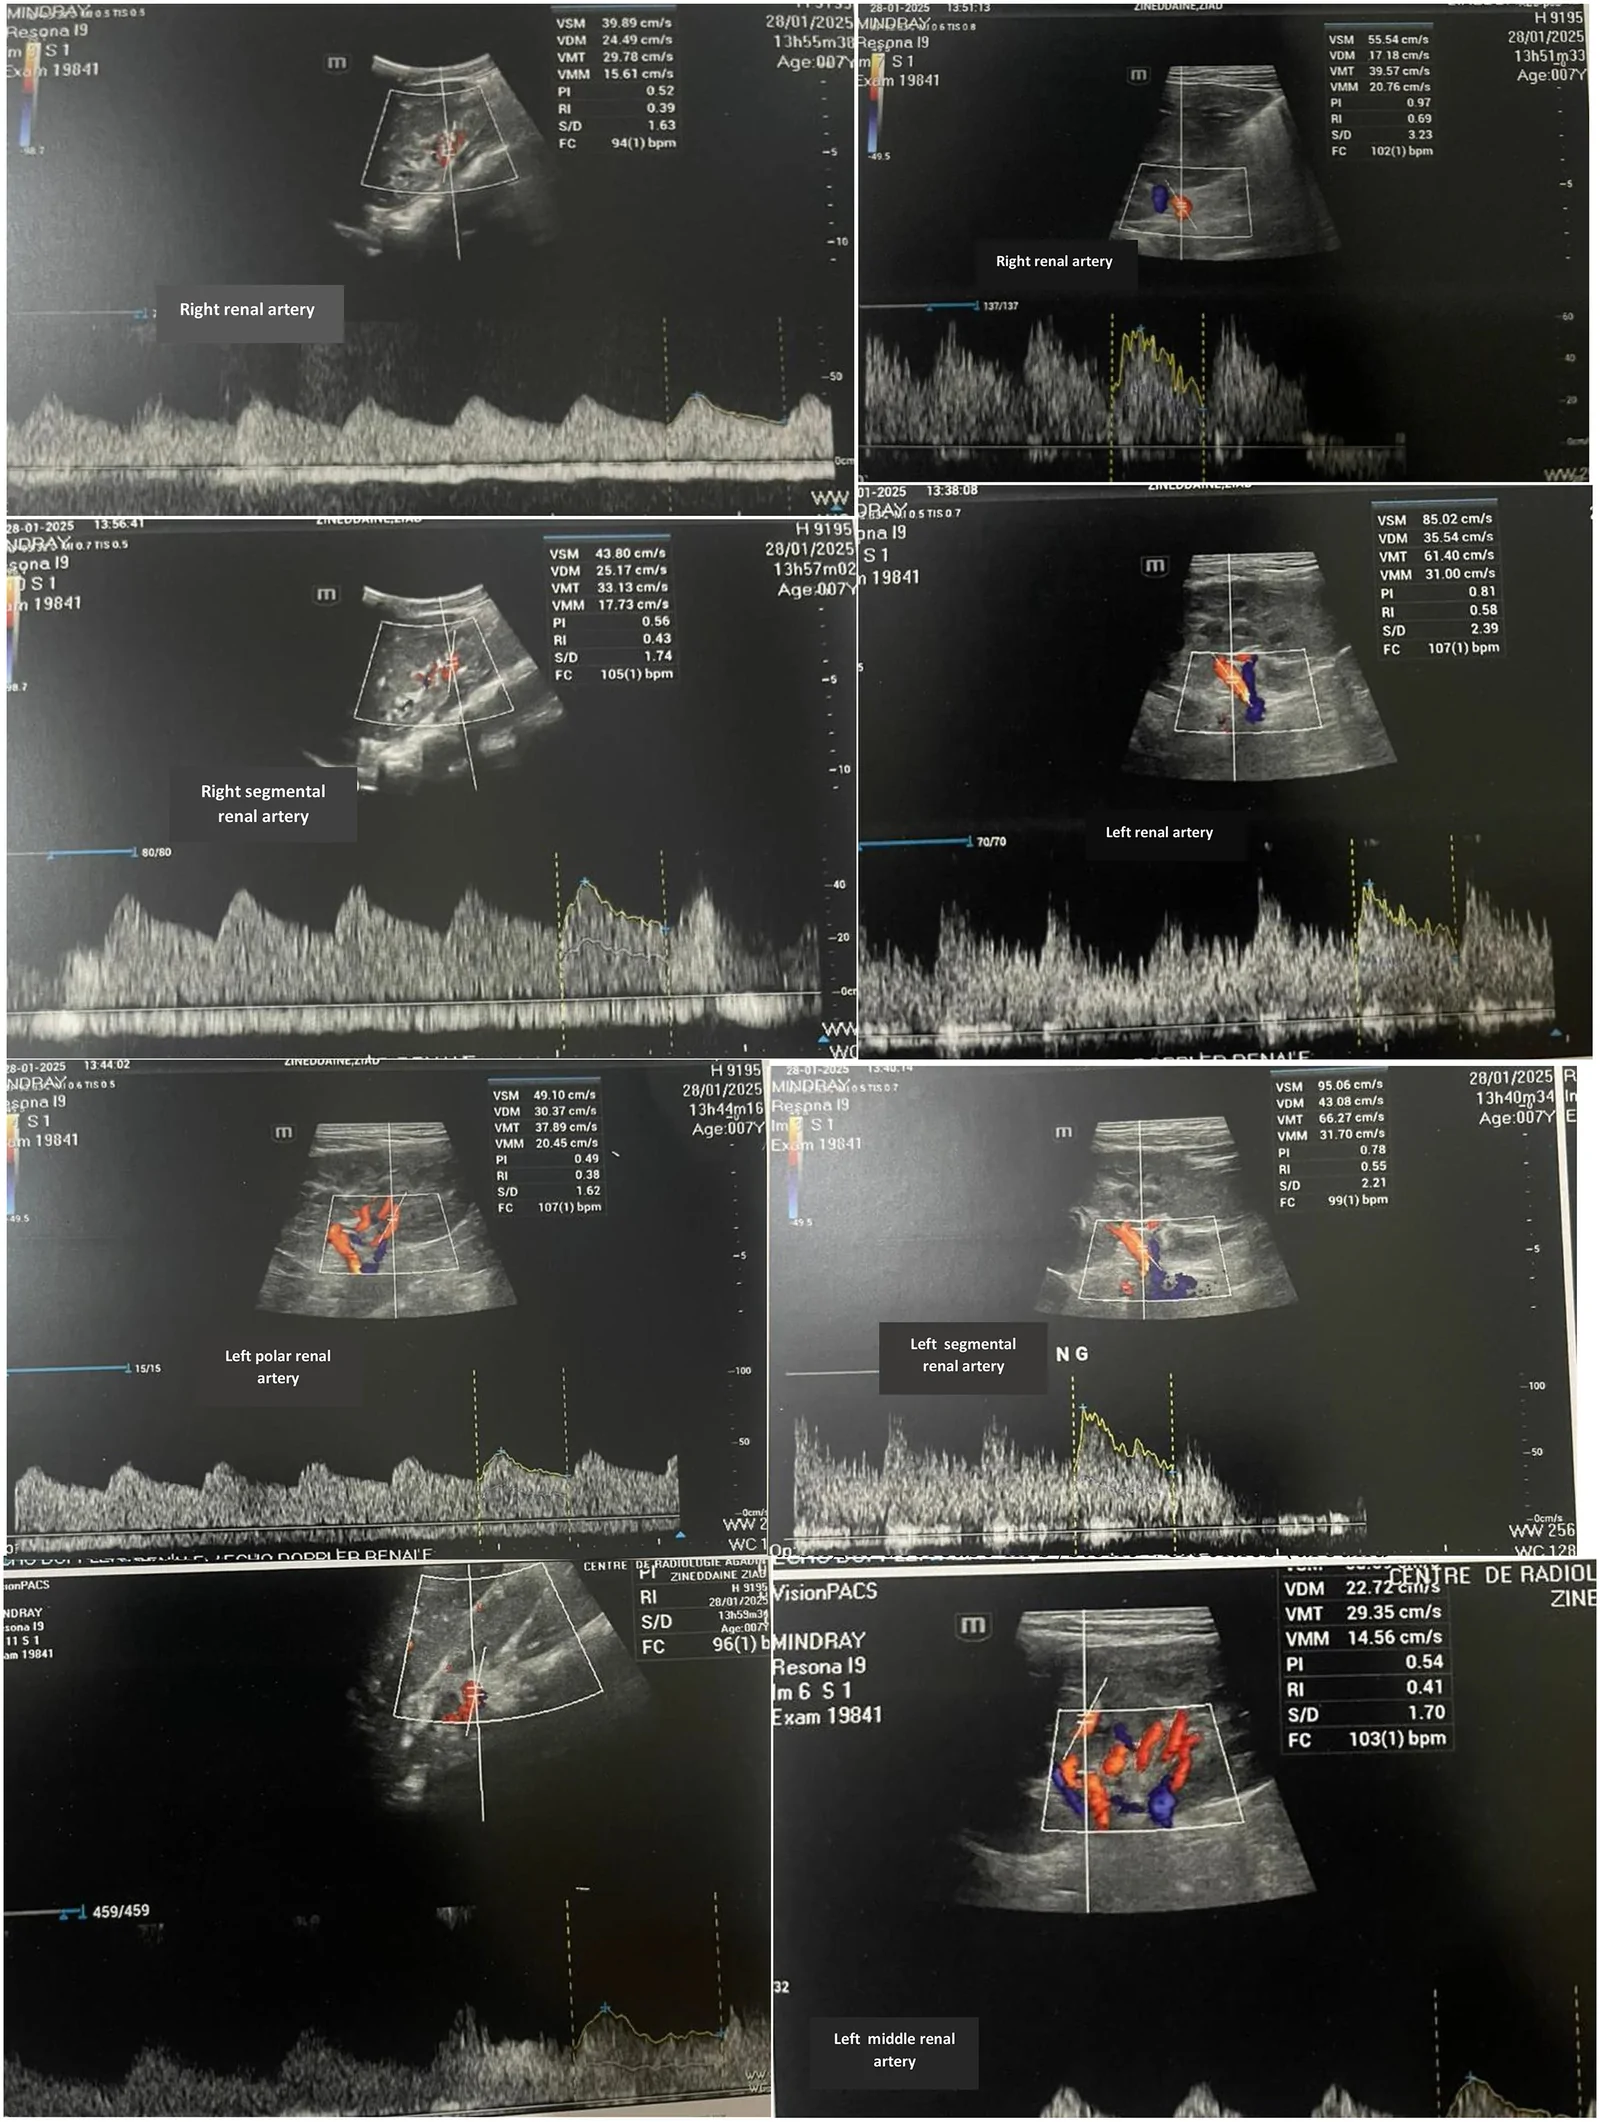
Color and spectral Doppler ultrasound examination demonstrated no measurable Doppler flow in the ostial and proximal segments of both renal arteries, consistent with severe bilateral proximal renal artery stenosis. Distal perfusion was preserved, with patent hilar, segmental, and interlobar renal arteries bilaterally. Intrarenal Doppler analysis revealed mildly reduced peak systolic velocities (approximately 50 cm/s) with preserved low resistive indices (RI: 0.53 on the right and 0.58 on the left). No prolongation of systolic acceleration time was observed, and no significant asymmetry between the 2 kidneys was detected.
